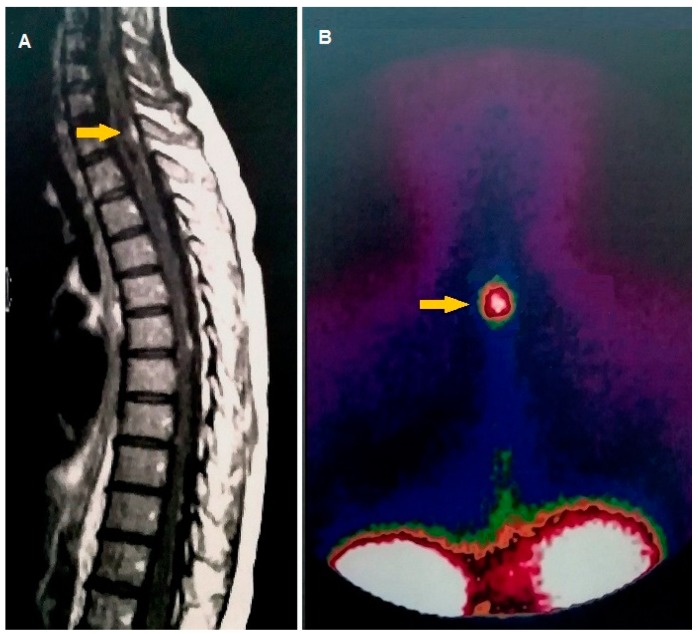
12-year-old female previously treated with surgery and chemotherapy for medulloblastoma of the posterior fossa. Sagittal contrast T1-weighted MRI shows nodular metastases of the spinal cord. The largest metastasis depicted by MRI (A, yellow arrow) was characterized by increased incorporation of [^111^In] pentetreotide at somatostatin receptor scintigraphy, as shown by posteroanterior planar projection (B, yellow arrow). The cerebrospinal fluid examination confirmed the diagnosis of leptomeningeal metastasis.
